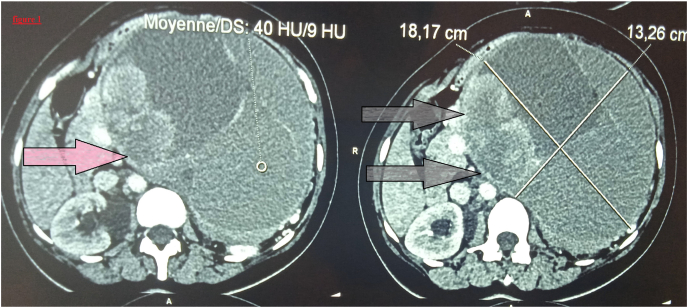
The computed tomography revealed a large left and paramedian intra peritoneal mass (arrow).
